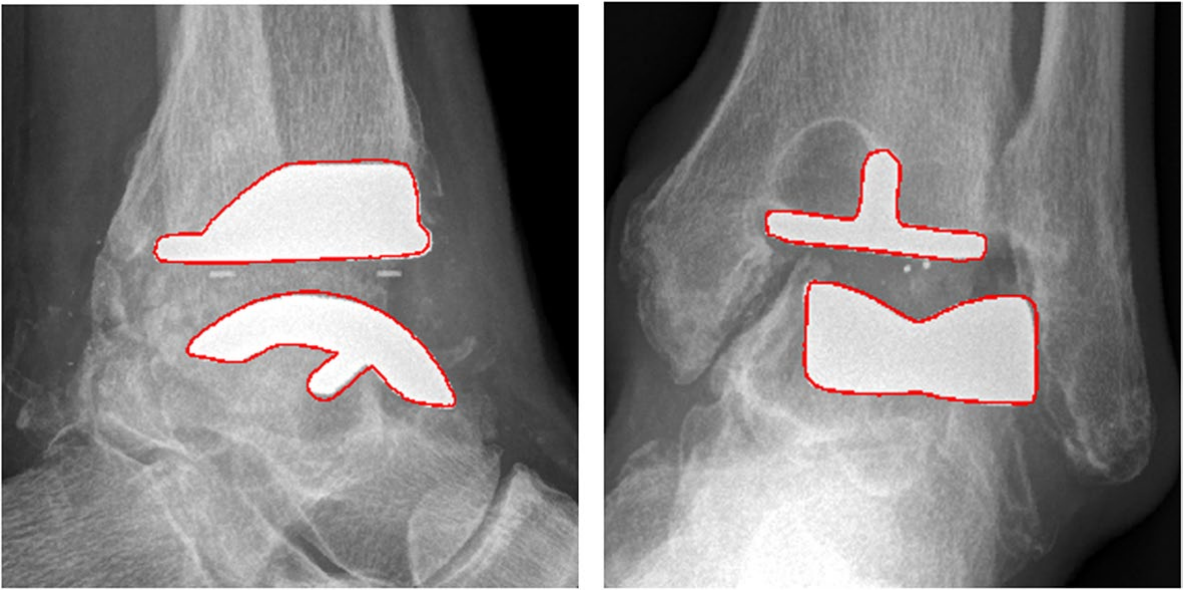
Contour mapping of the 3D TAR component contours on the lateral and anteroposterior radiographs
